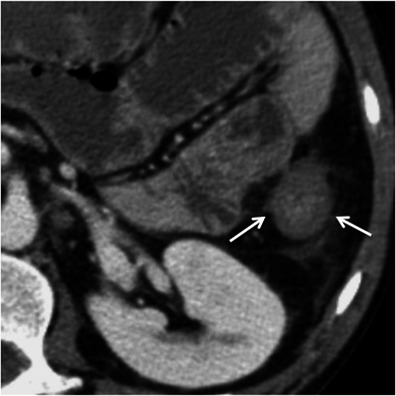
Well-differentiated adenocarcinoma of the descending colon. Axial contrast-enhanced CT scan showing focal concentric and regular thickening of the descending colon (*arrows*) with smooth contours and homogeneous enhancement. This proved to be a well-differentiated adenocarcinoma after biopsy. *Adapted from the electronic poster “Bowel wall thickening—a complex subject made simple” DOI:*10.5444/esgar2011/EE-063
